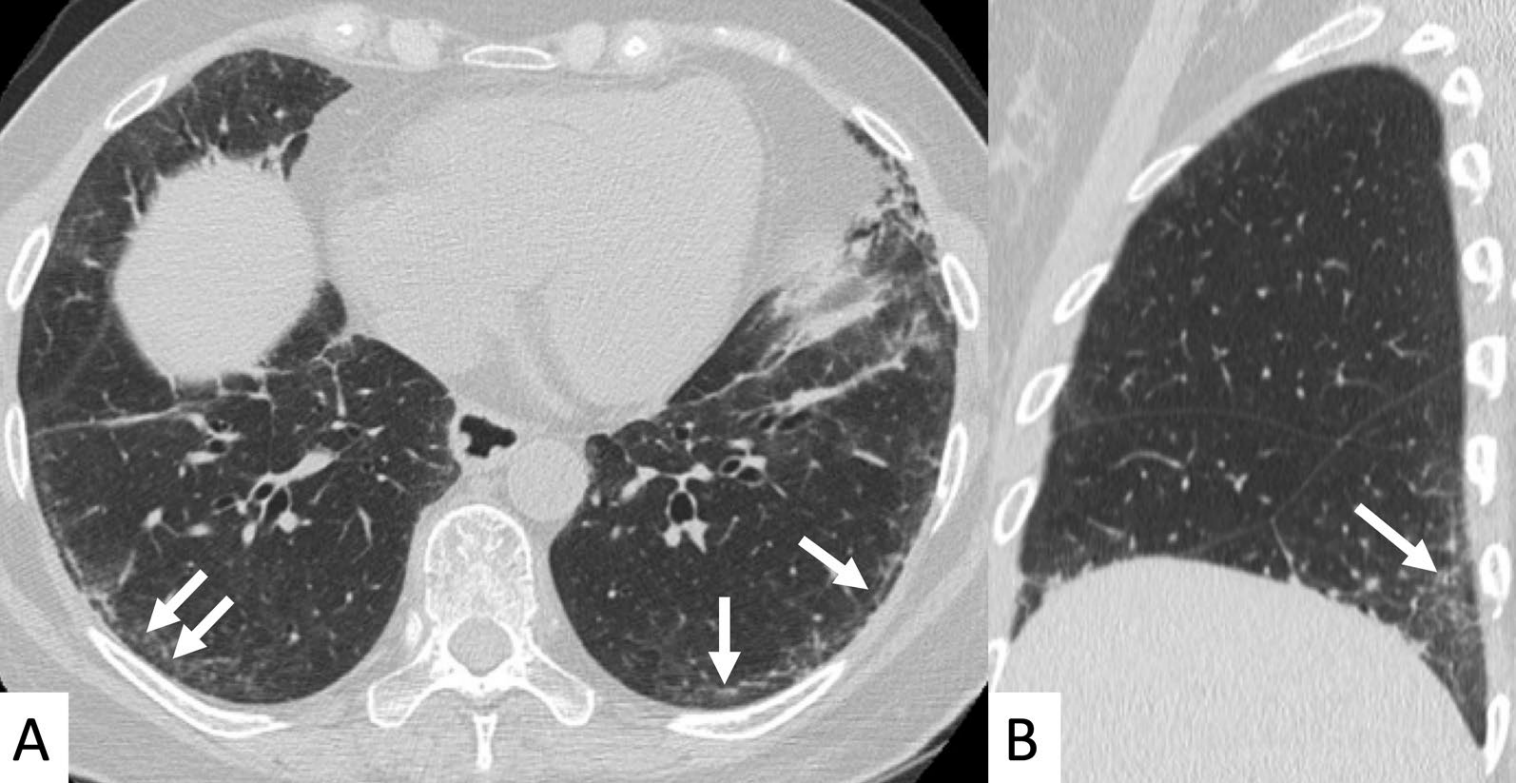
NSIP pattern with subpleural sparing in a female SSc patient. Axial (A) and sagittal (B) HRCT images demonstrate ground-glass opacities predominantly located in the basal pulmonary regions, with peripheral and subpleural distribution (white arrows). Pulmonary alterations may spare subpleural regions, as well demonstrated in A—namely in the left lower lobe
